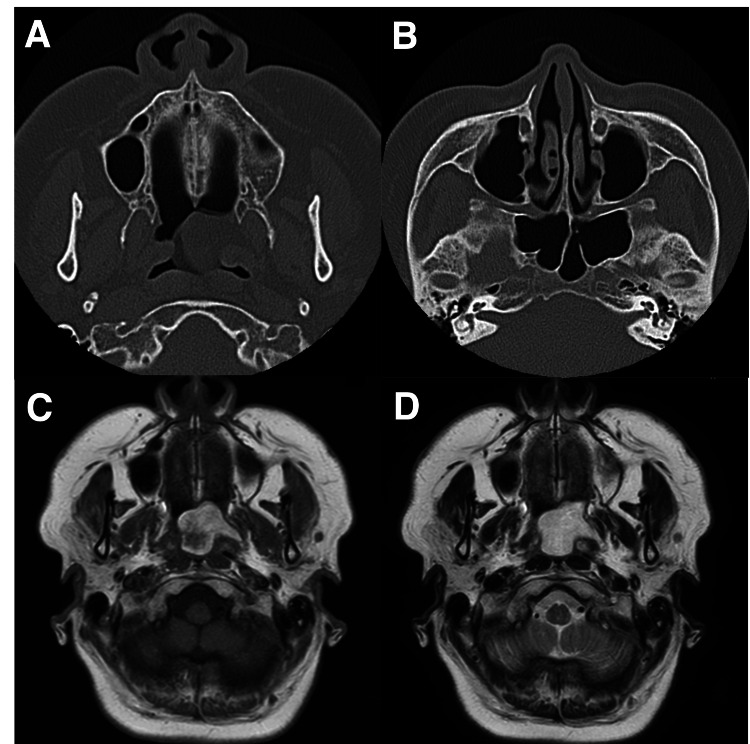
Findings of CT and MRI A: Axial plain CT scan revealed a tumor arising from the left eustachian tube with the same density as the surrounding fat. B: Axial plain CT scan revealed no evidence of OME. C: Axial plain T1-weighted MRI scan revealed a tumor with high signal intensity. D: Axial plain T2-weighted MRI scan revealed high signal intensity. CT: computed tomography, MRI: magnetic resonance imaging, OME: otitis media with effusion
